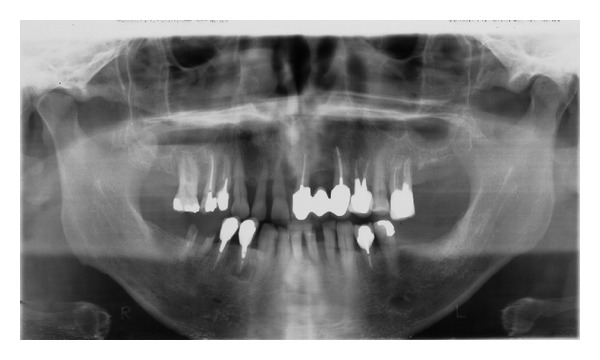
Radiographic image: panoramic radiography showing the extensive bone resorption in the anterior area of the maxilla compatible with advanced periodontal disease.
